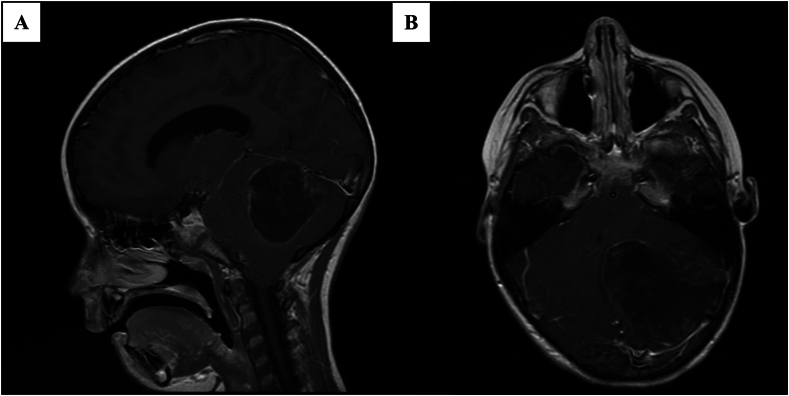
T1-Weighted sagittal (A) and axial (B) MRI images show a large mass occupying majority of the left cerebellar hemisphere. Small focal areas of necrosis are seen within the superior and lateral aspect of the mass. Some contrast enhancement of the margin of the mass is seen, however, the majority of the mass within the centre of the lesion does not show any significant enhancement. There is an inferior descent of the cerebellar tonsils with obliteration of the CSF spaces at the craniocervical junction.
